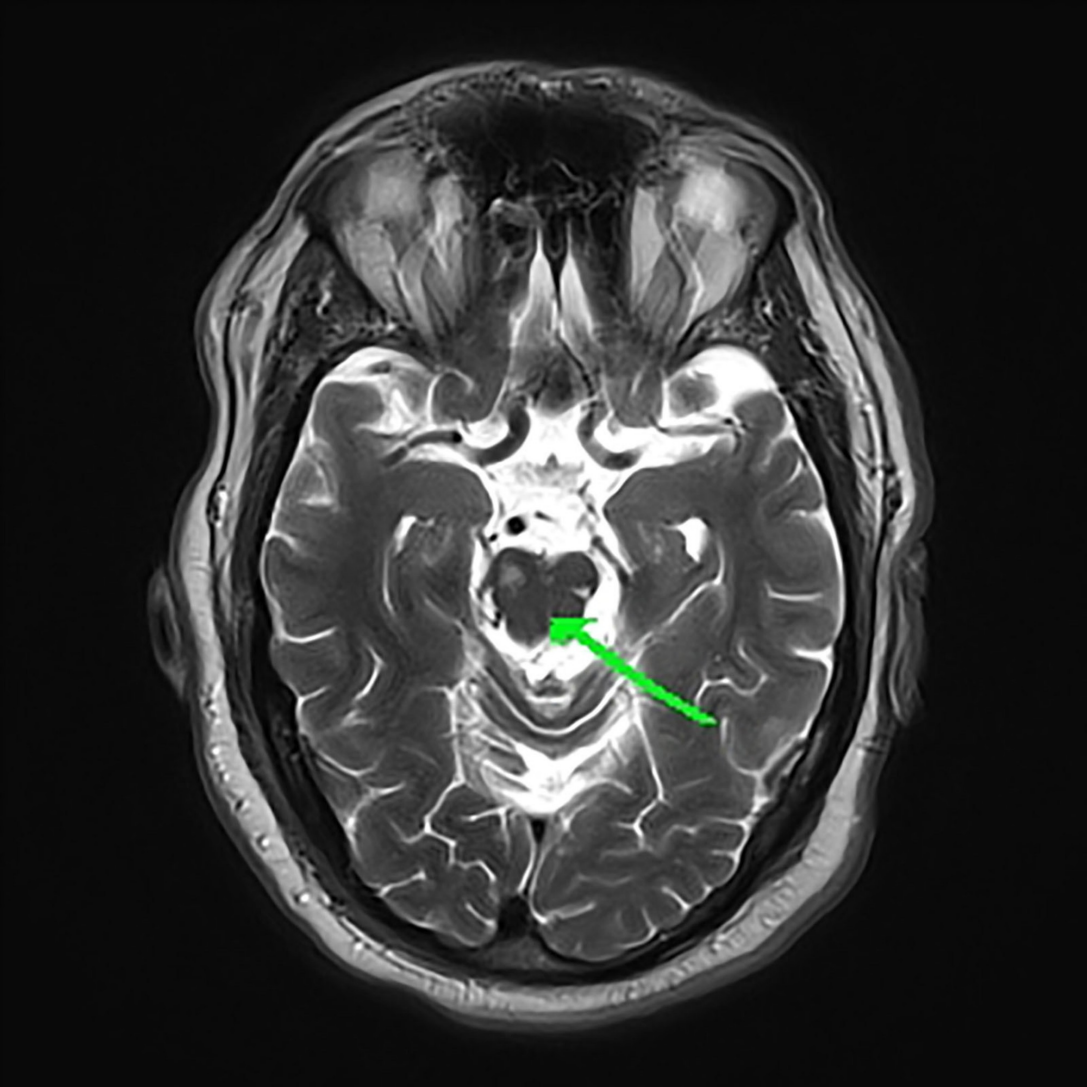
Magnetic Resonance Imaging (MRI) of the Brain Without Intravenous Contrast MRI of the brain shows a focus of subacute infarct involving the right side of the pons.
